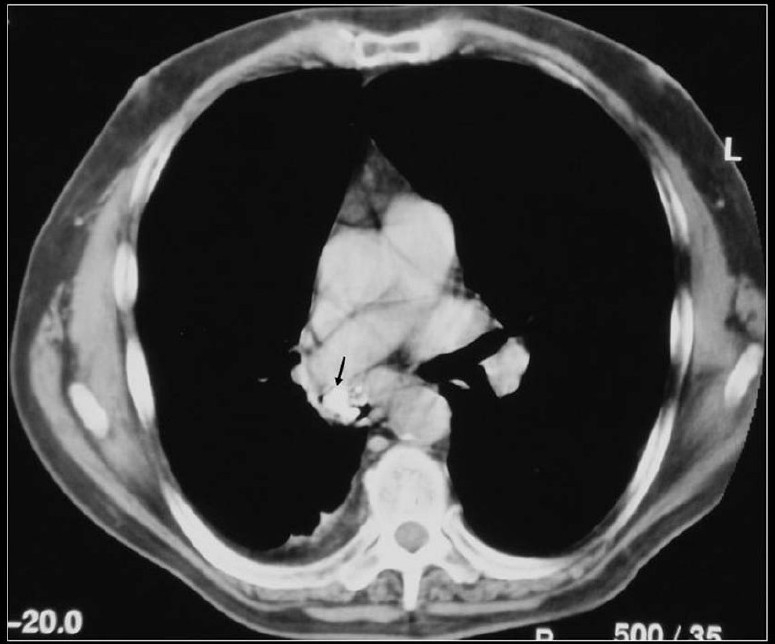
Axial CT scan shows a central pulmonary carcinoid associated with dense amorphous calcification (arrow)
